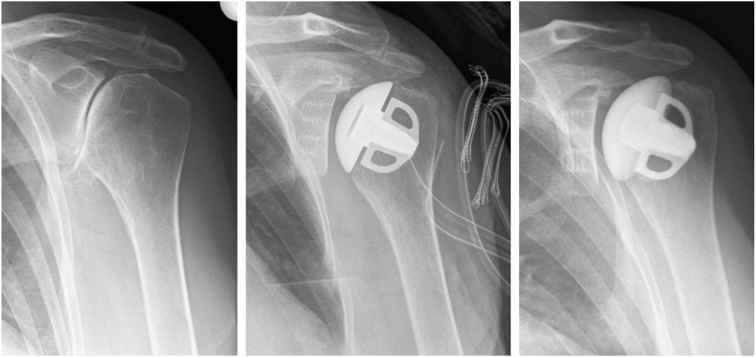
True ap radiographs of a 66-year-old female with primary osteoarthritis of the *left* shoulder undergoing aTSA with the Affinis Short stemless prosthesis and an Affinis Short Bionit ceramic head combined with an Affinis Glenoid vitamys uncemented glenoid component. Radiographs taken preoperatively, immediately postoperatively, and at 24 months postoperatively. *aTSA*, anatomic total shoulder arthroplasty.
